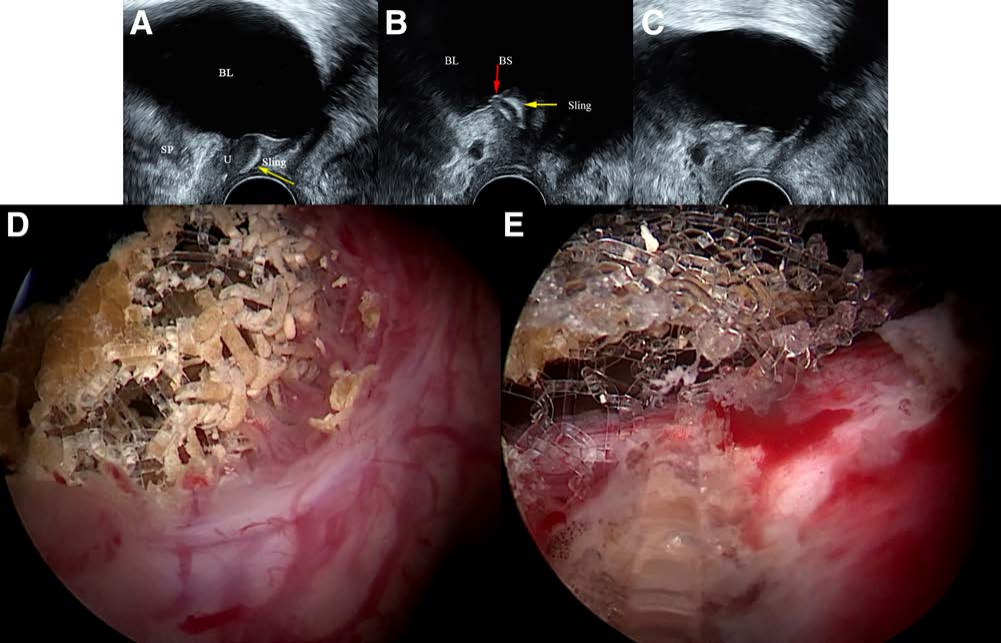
(A–C) Two-dimensional (2D) PFU images were obtained with the translabial method using a 3.5-MHz sector probe. Sagittal views were obtained at rest. The bladder (BL), bladder stones (BS), urethra (U), and sling were seen. The yellow arrows indicate the sling and the red arrow shows the bladder stones. (D and E) Images show mesh erosion into the urinary bladder and the formation of secondary bladder stones, which were resected using a holmium laser by cystoscopy. PFU = pelvic floor ultrasound.
